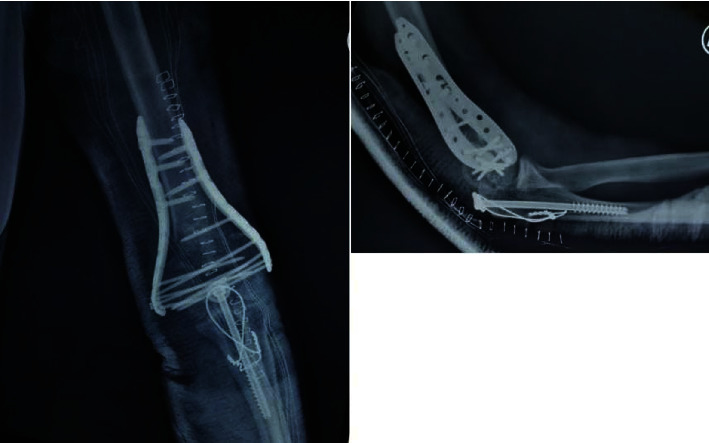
Postoperative radiographs of the elbow.
